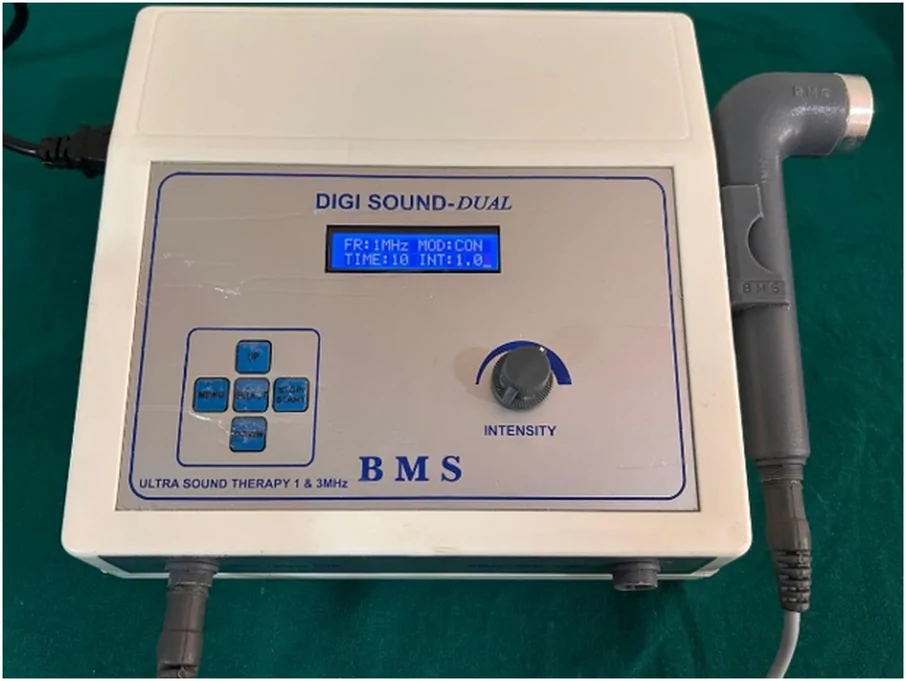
Therapeutic ultrasound with the parameters.
